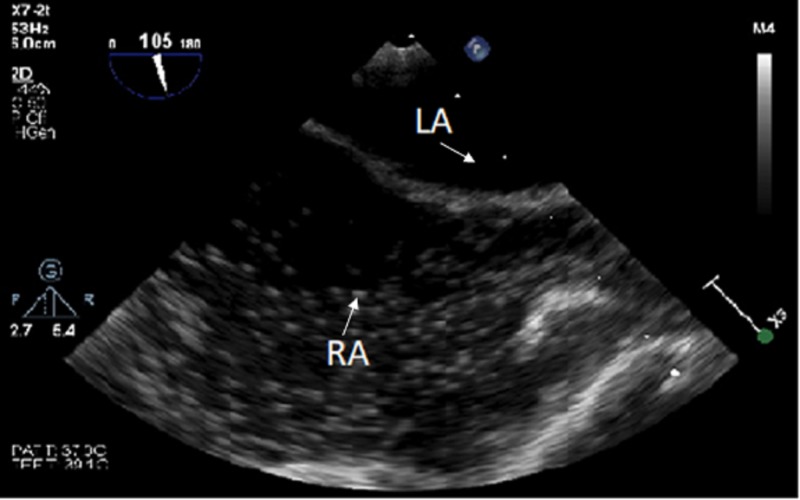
Pre-operative transesophageal echocardiogram, mid-esophageal bicaval view during agitated saline injection with Valsalva showing no evidence of intra-atrial shunt. RA: Right Atrium; LA: Left Atrium.
